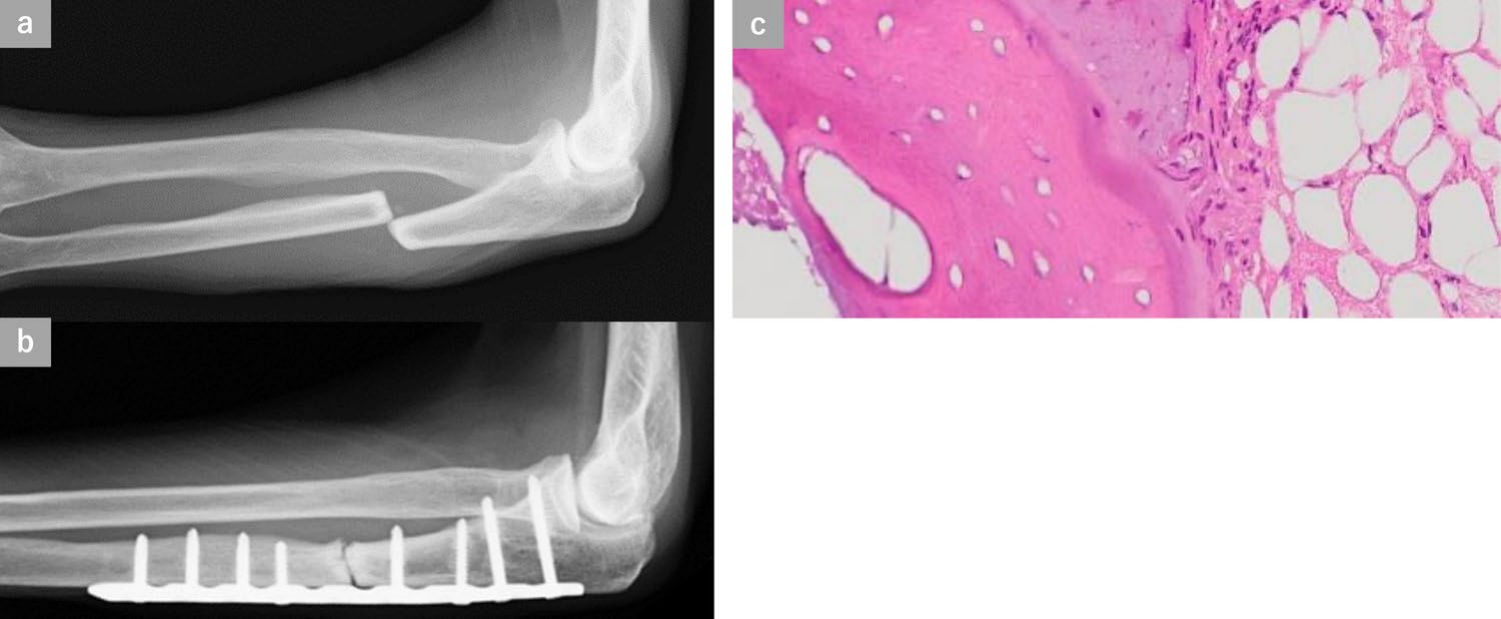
An 85-year-old woman (Patient No. 3) had a history of BP use for 7 years. When she was getting dressed, she noticed pain in her left forearm. Radiography revealed a transverse fracture with displacement of the proximal third of the ulna. The bone cortex around the fracture was thickened, the fracture showed osteosclerotic changes, and the medullary canal was closed, indicating a Stage III AUF (a). Surgery was performed using a straight locking plate (SLP) (LCP metaphyseal plate; DePuy Synthes Warsaw, IN) (b). Pathological findings of bone tissues collected from the fracture site revealed that there were no osteoblasts or osteoclasts on the surface of the trabecular bone, suggesting the suppression of bone turnover (c)
